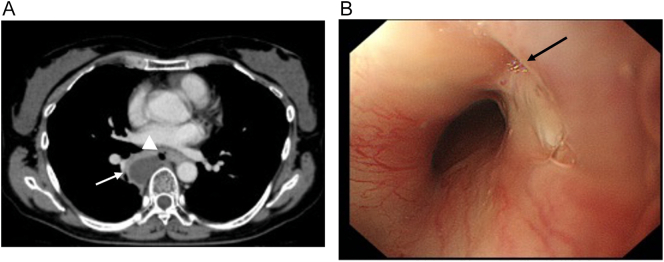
Preoperative findings. A) Contrast-enhanced computed tomography scan showed a 30 × 25 mm encapsulated fluid retention in the mediastinum (closed arrow) and a bubble near the esophagus (crossed arrowhead). B) Upper gastrointestinal endoscopy revealed a fistula at the 2 o'clock position, 30 cm from the incisor (closed arrow).
